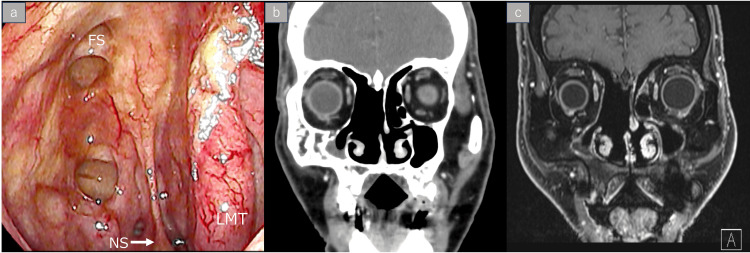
Disease status at six-year follow-up. Endoscopic and imaging findings demonstrated no evidence of disease recurrence six years after treatment. A residual nasal septal perforation, likely attributable to surgical and chemoradiation effects, was observed. (a) Nasal endoscopy showing a residual septal perforation. (b) Coronal contrast-enhanced CT. (c) Coronal contrast-enhanced MRI. NS: nasal septum; LMT: left middle turbinate; FS: frontal cell
